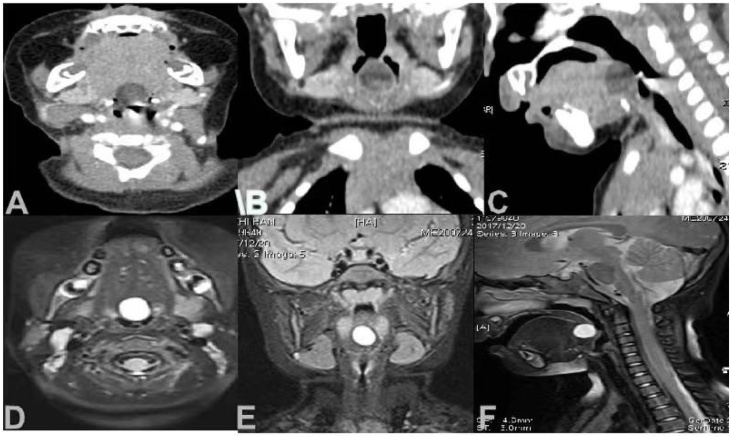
(A, B, C) CT of larynx. A round cyst of low density can be seen at the base of the tongue. (D, E, F) MRI of larynx. A round cyst can be seen at the base of the tongue.
